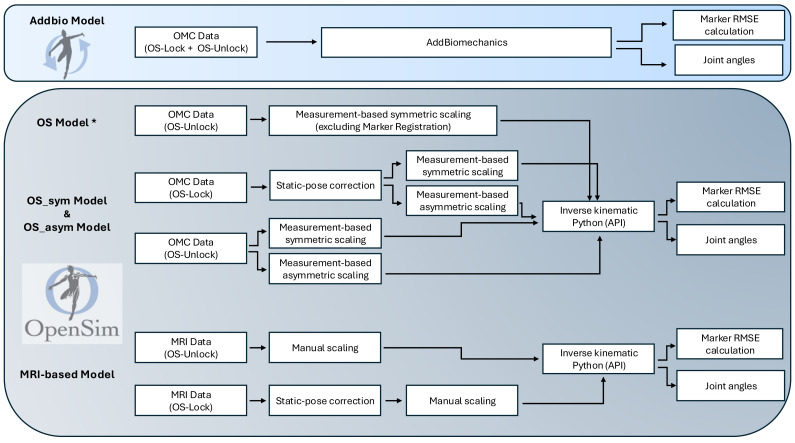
An overview of the different steps in the process of generating the five distinct personalized models. Addbio Model is created with AddBiomechanics and OS_sym Model (symmetric), OS_asym Model (asymmetric) and MRI-based Model are created with OpenSim. The Armless Rajagopal model was used as the standard generic model in all approaches. The gait data is divided into OS-Lock and OS-Unlock, based on the locking of certain joints before scaling in OpenSim.
